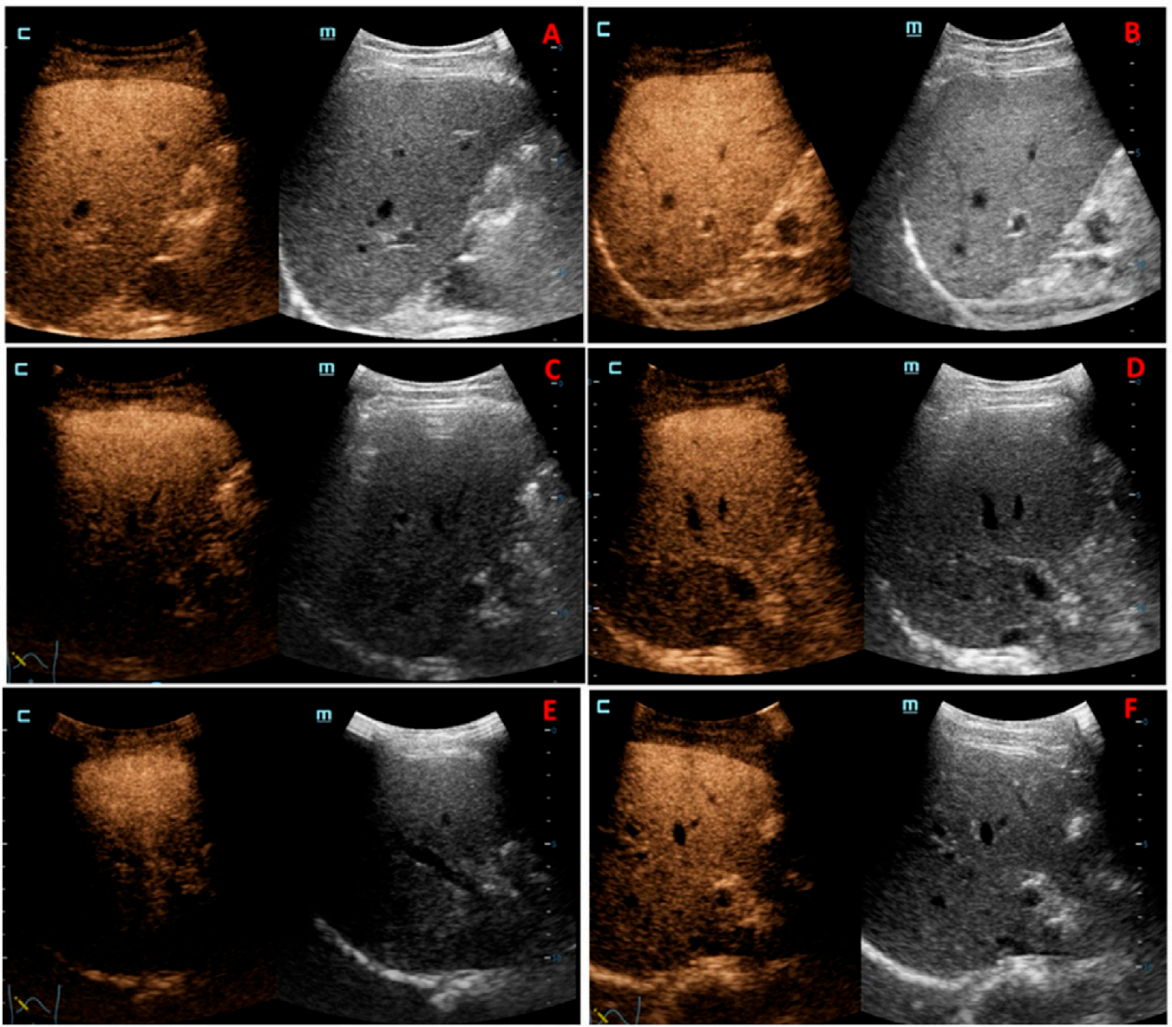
Ultrasound images at 5 min (A,C,E) and 20 min (B,D,F) after administration in the low-dose group, medium dose group, and high-dose group.
